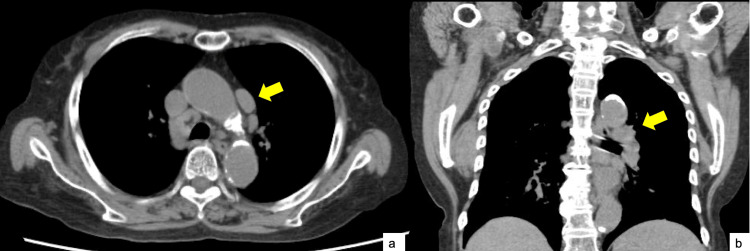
Results of plain chest computed tomography (CT) a: CT in the axial plane indicating mediastinal lymphadenopathy. b: CT in the coronal plane indicating hilar lymphadenopathy.
